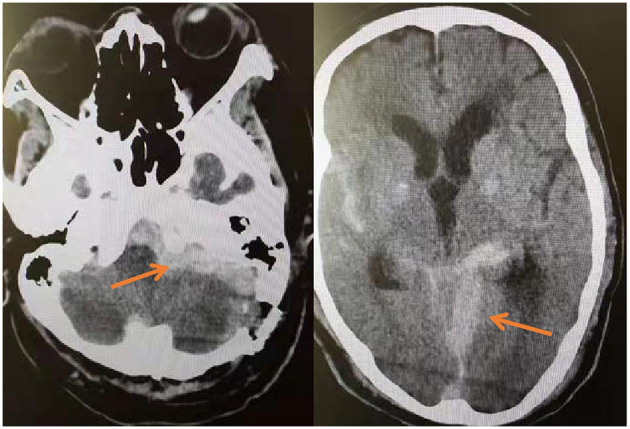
On the third day after MVD, the patient's blood pressure increased intermittently, and the corresponding antihypertensive treatment was given. On the fourth day, the patient suddenly vomited with progressive disturbance of consciousness. The reexamination of head CT showed the operation area and subarachnoid hemorrhage.
